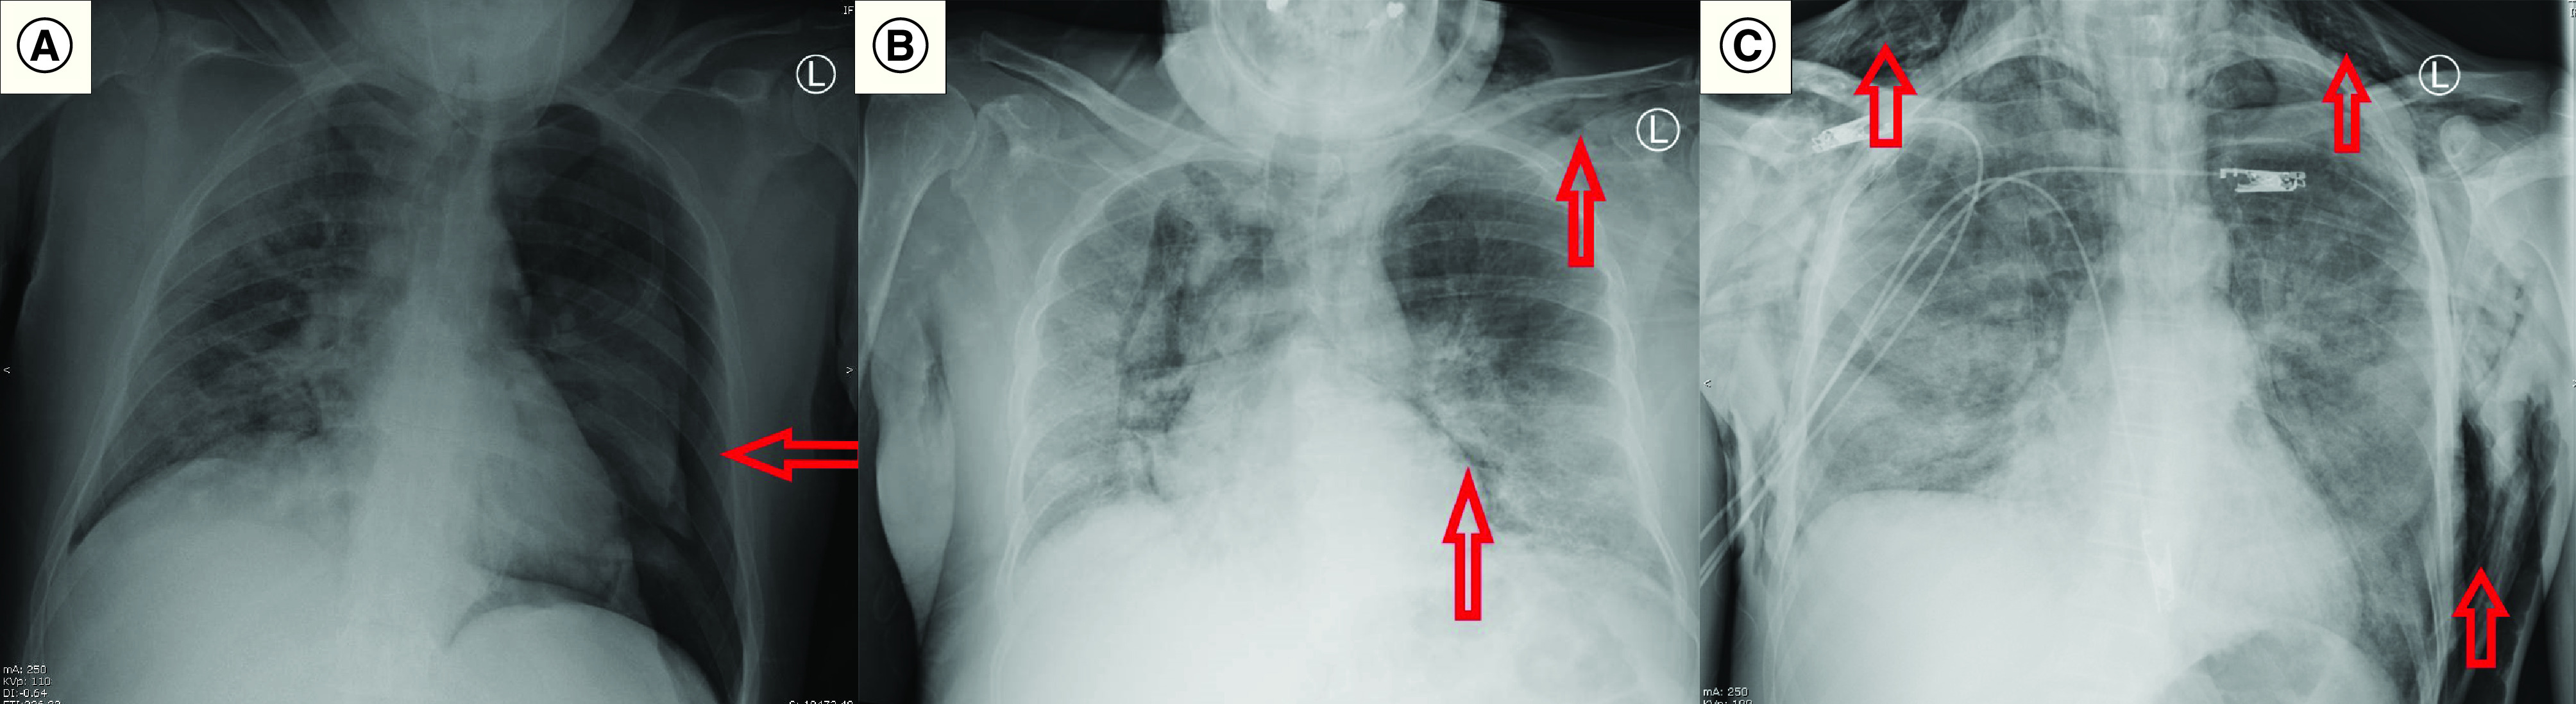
Radiological findings of pneumothorax, pneumomediastinum and subcutaneous emphysema. X-ray images showing: (A) PNX in Case #2. (B) PMD and SCE in Case #5. (C) SCE in Case #15. PMD: Pneumomediastinum; PNX: Pneumothorax; SCE: Subcutaneous emphysema.
